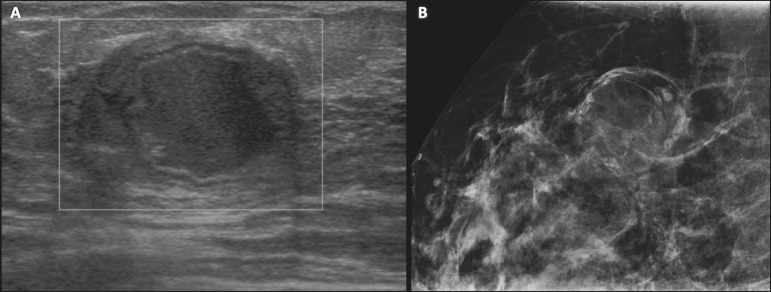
A 29-year-old lactating patient complained of a palpable mass in the right breast. Ultrasound (A) showed a complex cyst with hypoechoic content and thickened walls, with no significant flow on the Doppler study. Mammography (B) showed a nodule with peripheral calcifications and areas of fat density within it. FNAB confirmed the diagnosis of galactocele.
